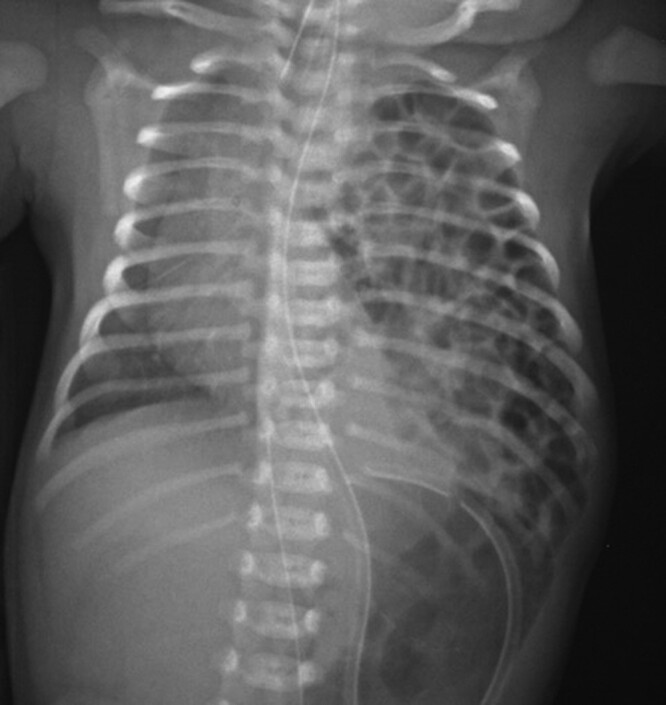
Plain radiograph of a newborn with left congenital diaphragmatic hernia (CDH)
